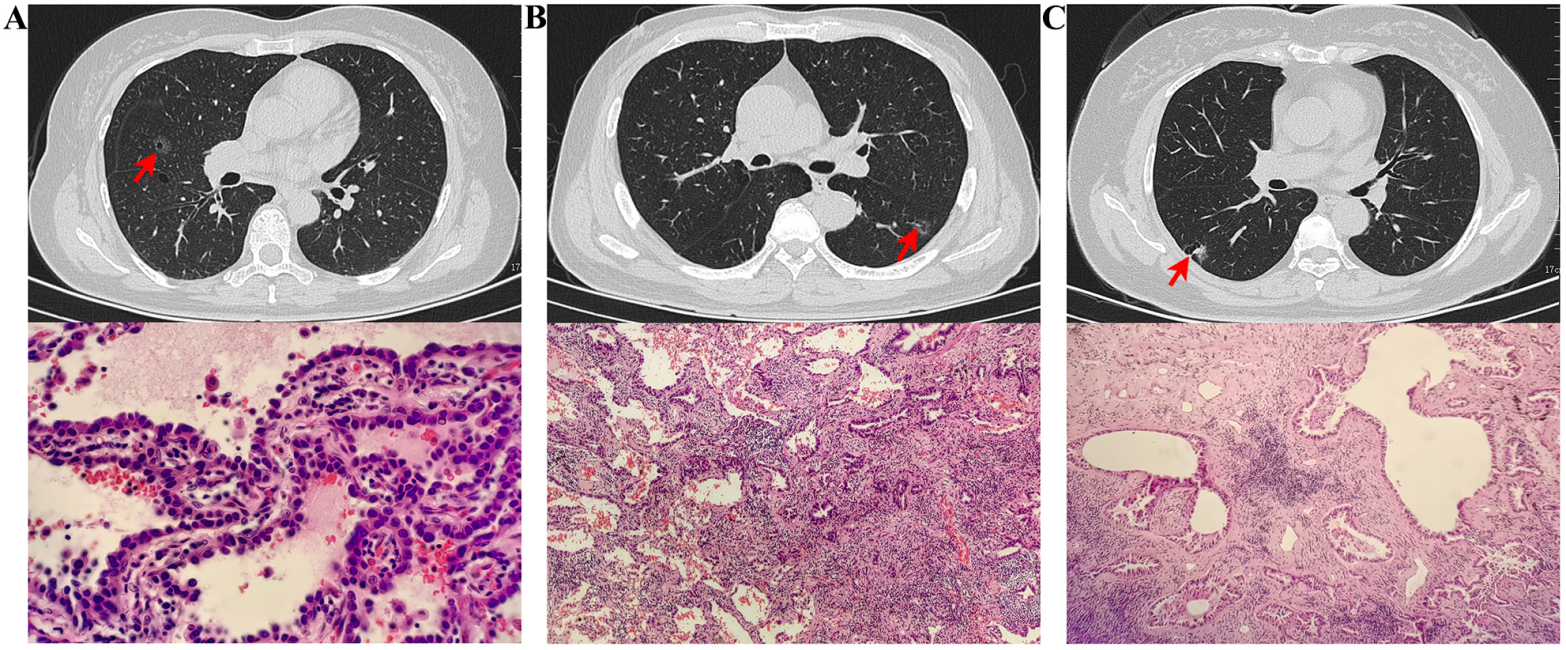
Radiologic and pathologic features of LCCA: a comparison of adenocarcinoma in situ (A), Minimally Invasive Adenocarcinoma (B), and Invasive Adenocarcinoma (C). (A) CT scan revealed a ground-glass nodule in the middle lobe of the right lung, with a small lucent area inside. Pathological findings showed cancer cells growing in a single layer along the alveolar walls, with dense cell arrangement but no vascular or lymphatic invasion. (B) CT scan revealed a ground-glass nodule in the lower lobe of the left lung, with visible vacuoles and lobulation. Pathological findings showed most cancer cells growing along the alveolar walls, with focal stromal fibrosis and visible acinar structures, measuring less than 5 mm in size. (C) CT scan revealed a cavitary nodule in the lower lobe of the right lung, with pleural traction nearby and traversing blood vessels inside. Pathological findings indicated invasive growth of cancer tissue with acinar structures.
